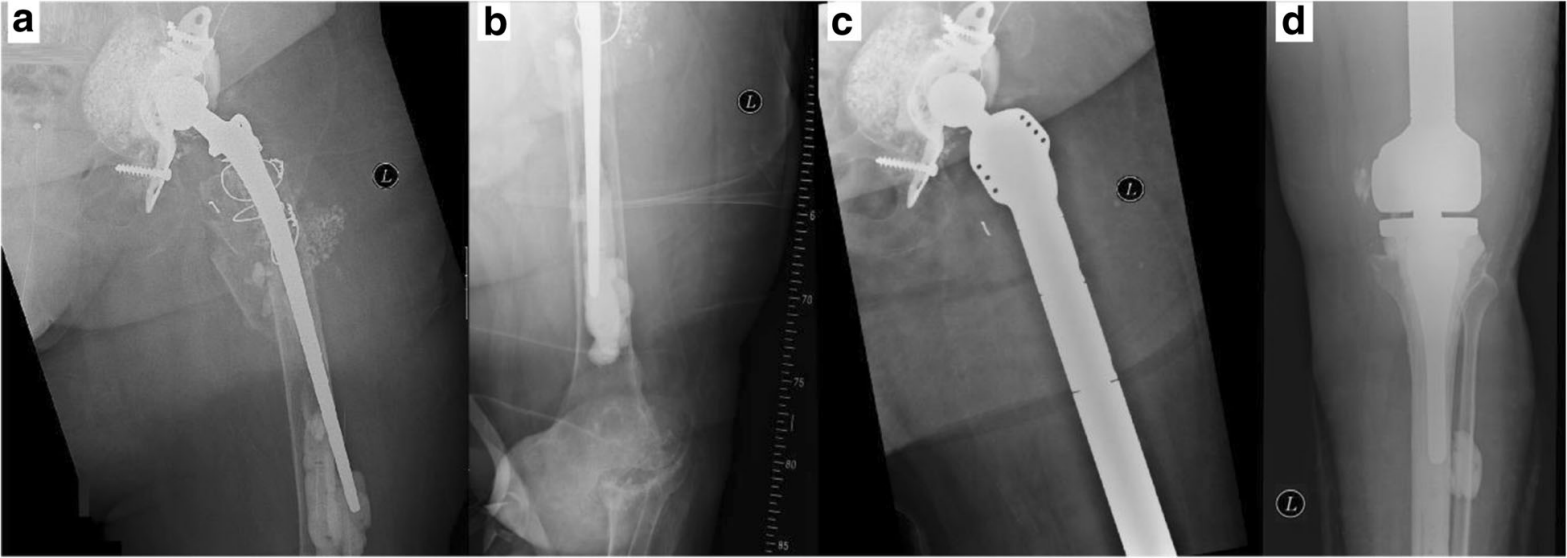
a, b X-ray of a patient with periprosthetic fracture at the level of the left femur and concomitant destructive osteoarthritis of the knee. c, d Reconstruction of femoral integrity was performed with total femoral replacement with MML-Prosthesis (acetabular cup was left in place due to no signs of loosening)
